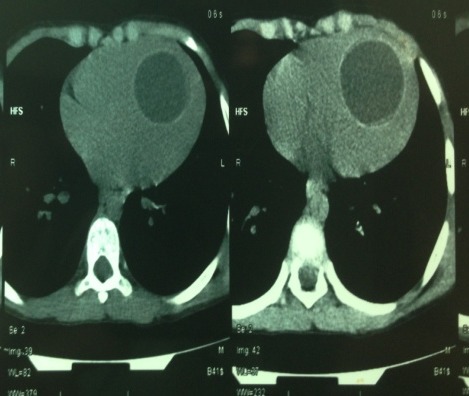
CT Scan: a hypodense round liquid formation in the left ventricle
